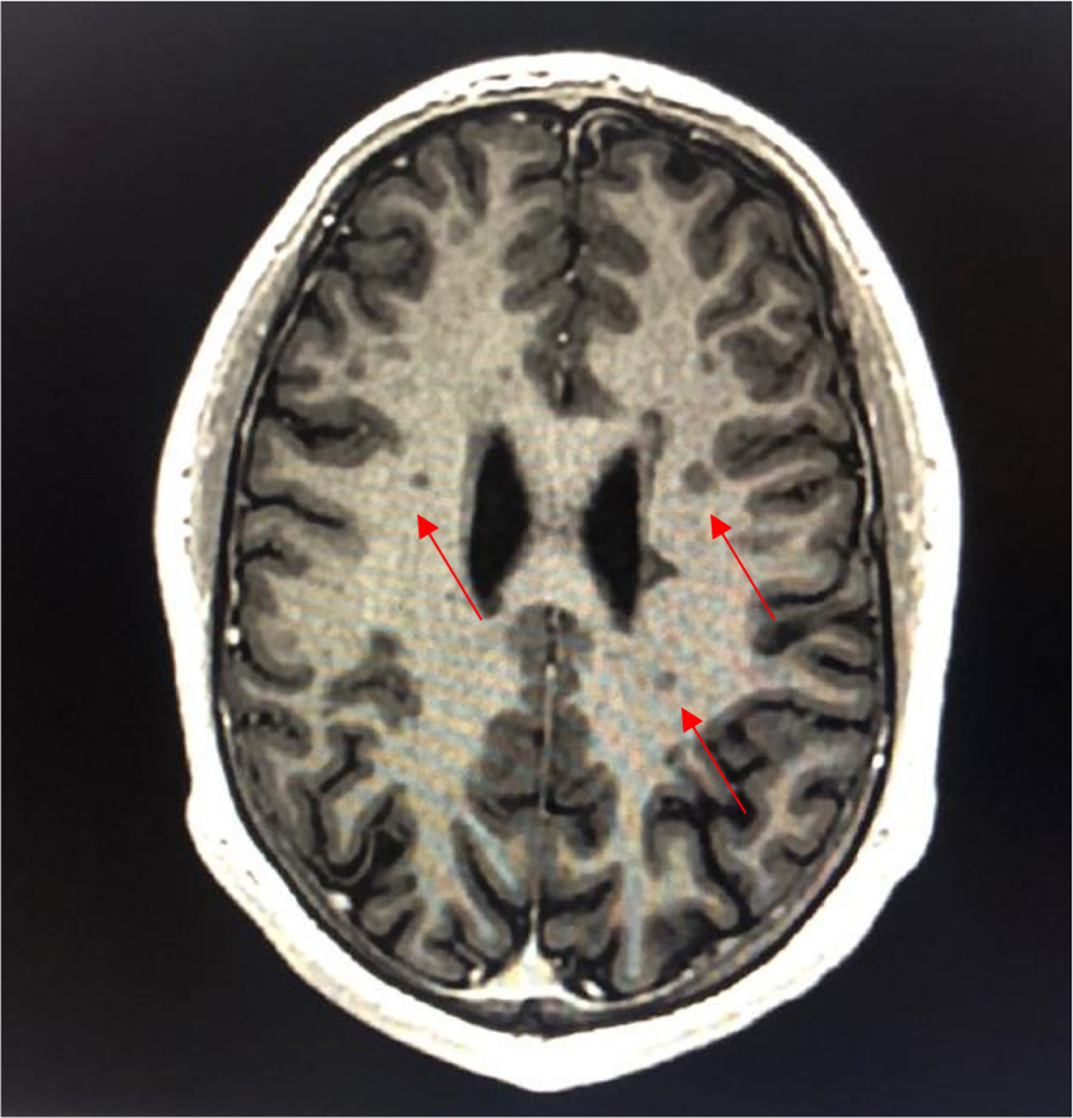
T1 MRI of the brain in December year X+1 showing black holes.
